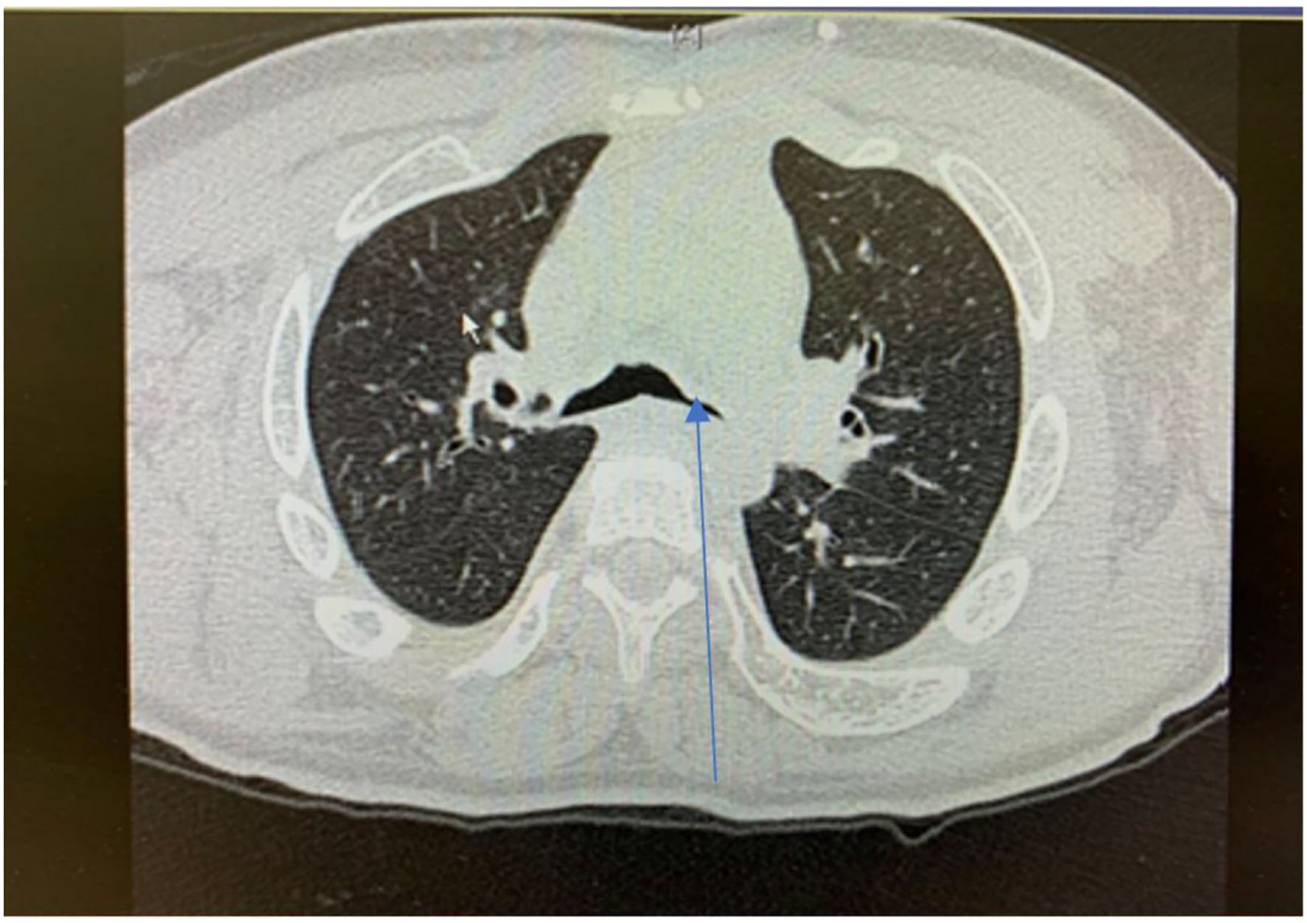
Cross sectional thoracic CT scan shows bronchomalacia in adult MPS IV.
